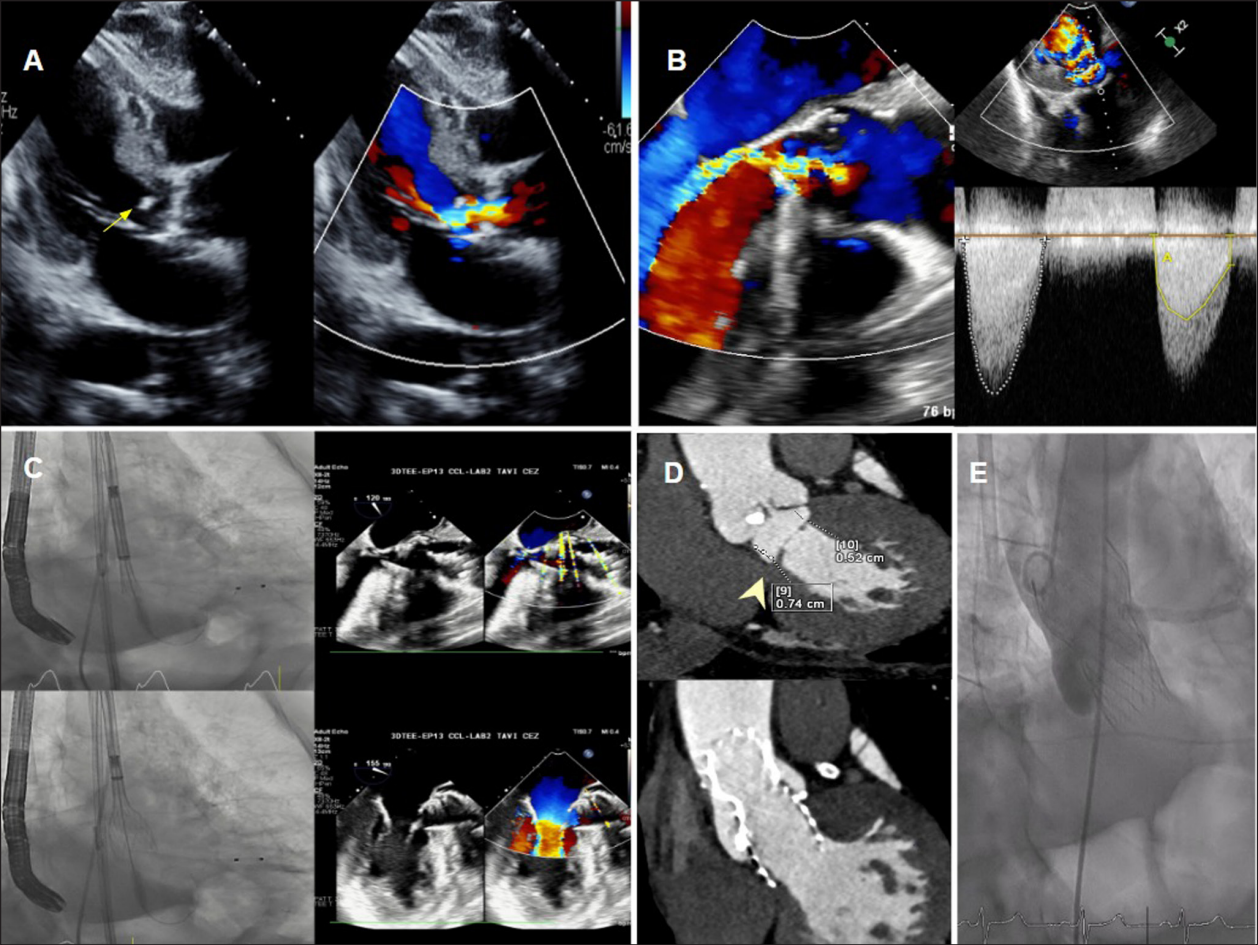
(A) Transthoracic echocardiography parasternal long-axis view showing subaortic membrane (arrow) with flow acceleration in the left ventricular (LV) outflow tract and below the aortic valve. (B) Transesophageal echocardiography (TEE) long-axis view showing subaortic membrane with flow acceleration in the LV outflow tract and below the aortic valve, continuous-wave Doppler showing a double-envelope pattern. The inner envelope on the right side of the image (yellow) represents stenosis at the subaortic membrane, while the outer envelope on the left side of the image (white) represents stenosis at the aortic valve. (C) A 26-mm Evolut™ PRO+ transcatheter aortic valve implantation (TAVI) was deployed with TEE guidance to position the valve precisely at the subvalvular ridge. (D) Cardiac computed tomography (CT) multiplanar reconstruction shows a subaortic membrane (arrowhead), and a 1-year post-TAVI CT demonstrates a well-seated valve covering most of the left ventricular outflow tract (LVOT), including the previously identified subaortic membrane. (E) Final aortography shows a well-seated valve in the LVOT covering the subaortic membrane, with no paravalvular leak.
